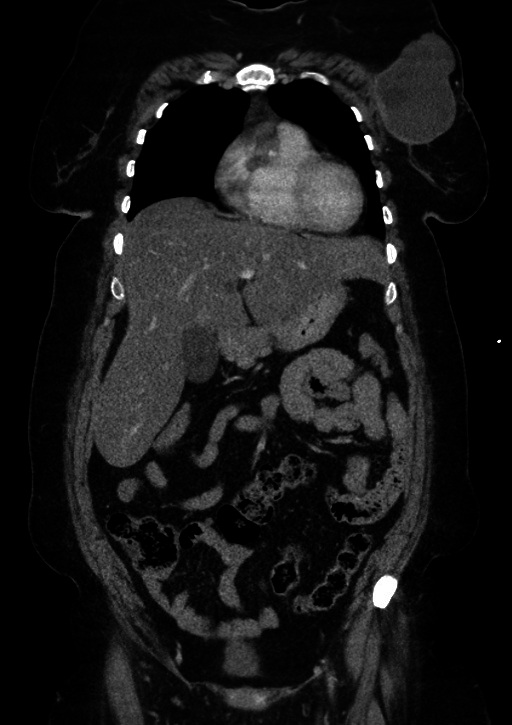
Coronal CT image shows the left breast mass with internal hypo dense cystic component
